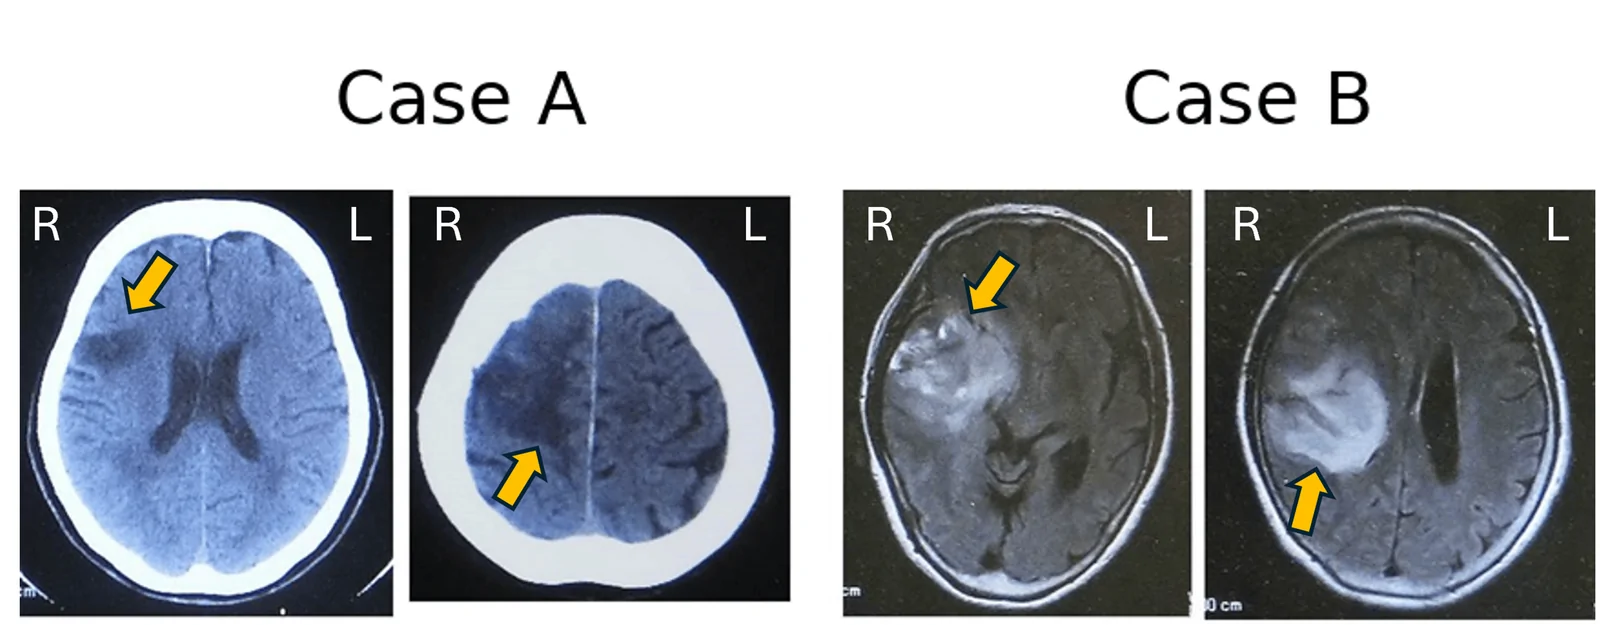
Neuroimaging findings in the two cases Representative axial brain images demonstrating right hemisphere lesions in Case A (computed tomography) and Case B (magnetic resonance imaging).
